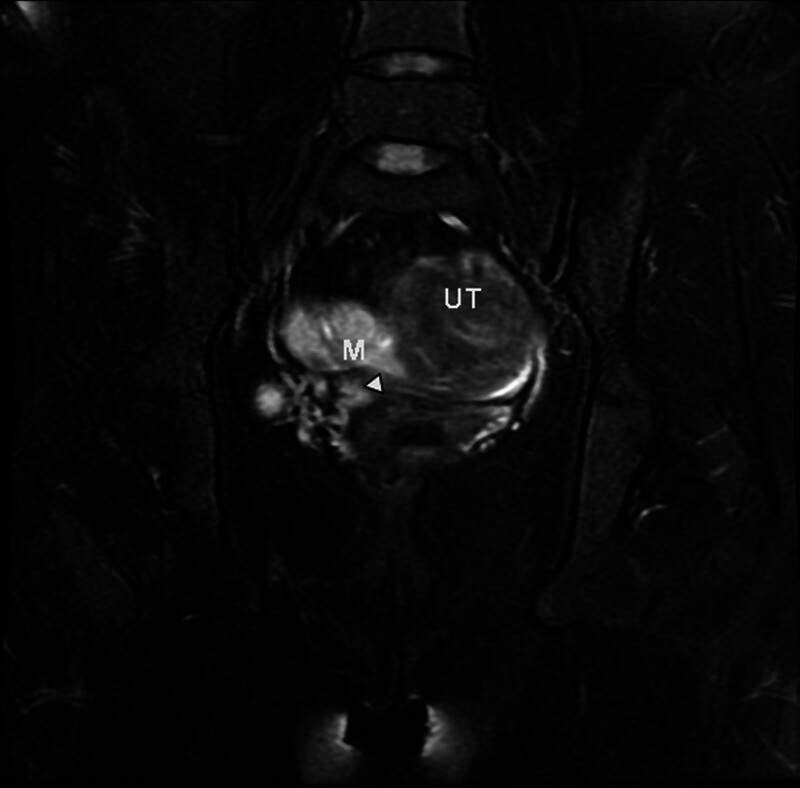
Magnetic resonance imaging (MRI) coronal section demonstrated a right parametrial mass of adnexal origin with myometrial invasion (arrow indicates herniated segment of the ipsilateral fallopian tube within the uterine musculature). UT = uterus, M = mass.
